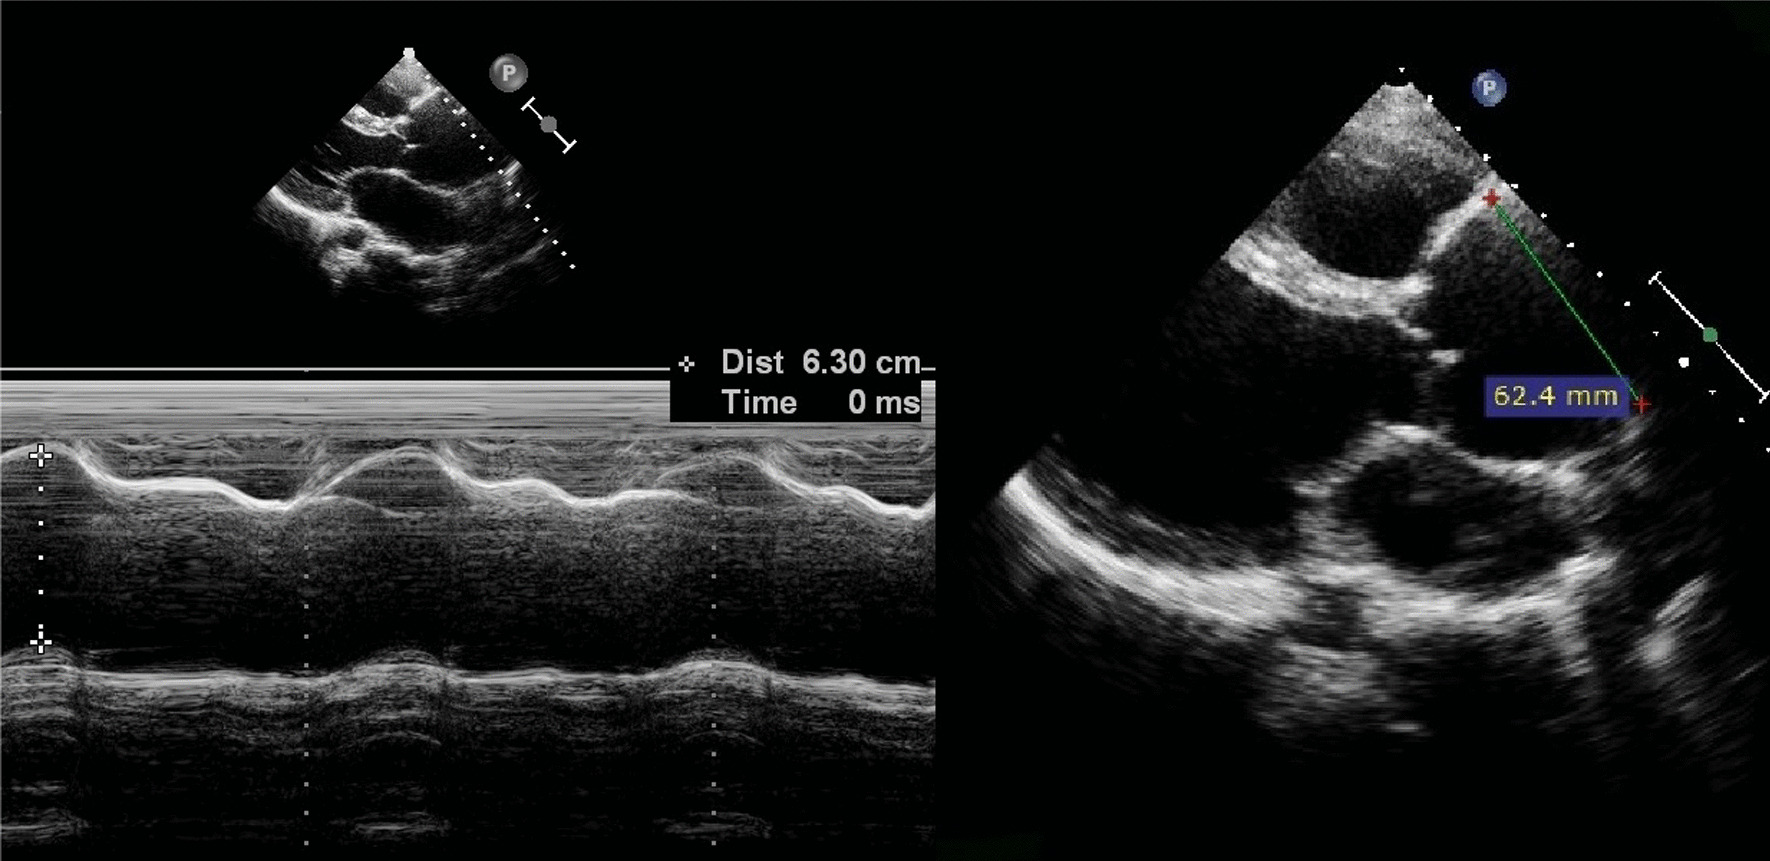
Echocardiogram/M-mode and 2D measurements of ascendent aorta
